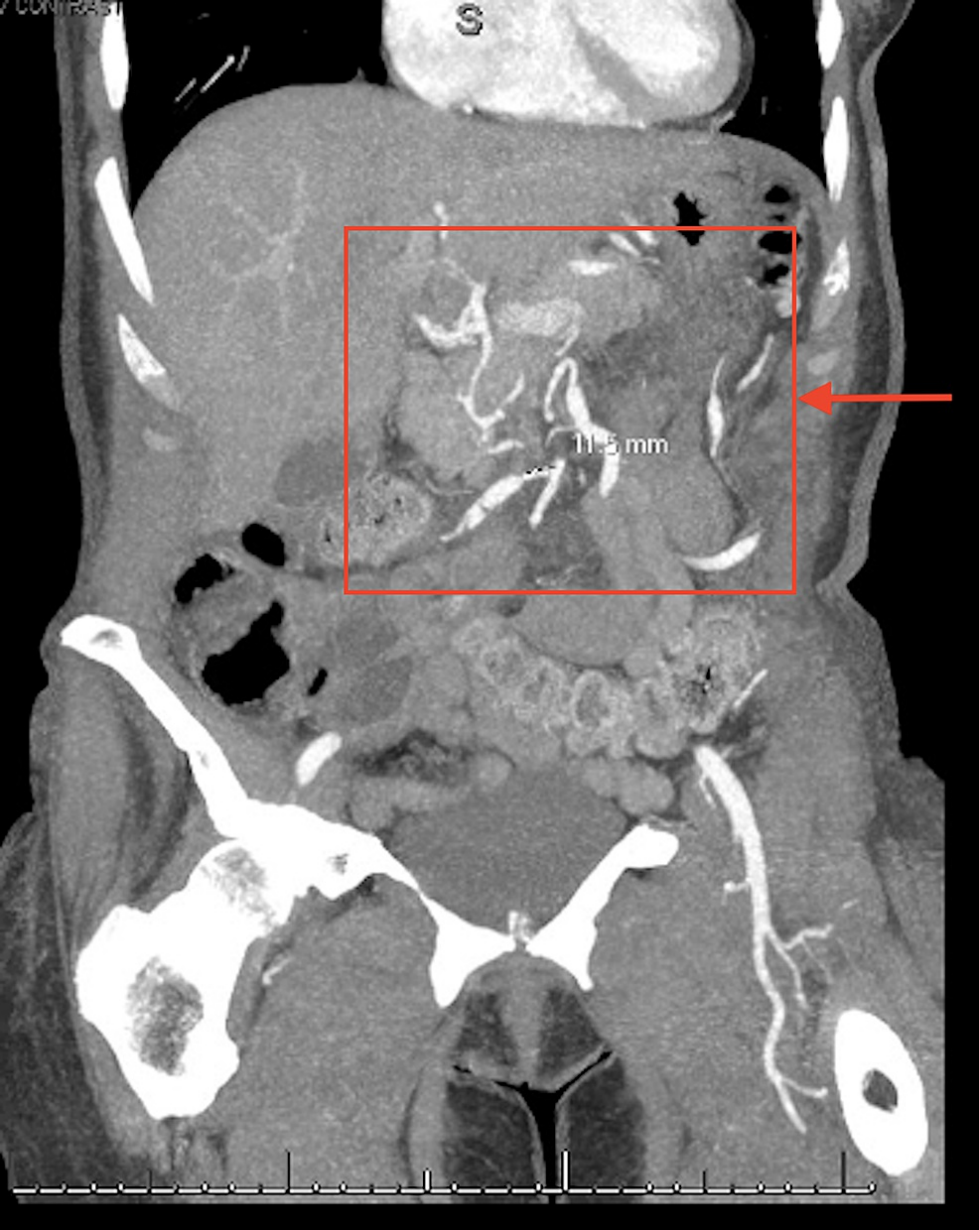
Coronal CTA demonstrating multifocal areas of SMA and IMA branch narrowing and dilation (arrow). CTA: computed tomography angiography, SMA: superior mesenteric artery, IMA: inferior mesenteric artery.
